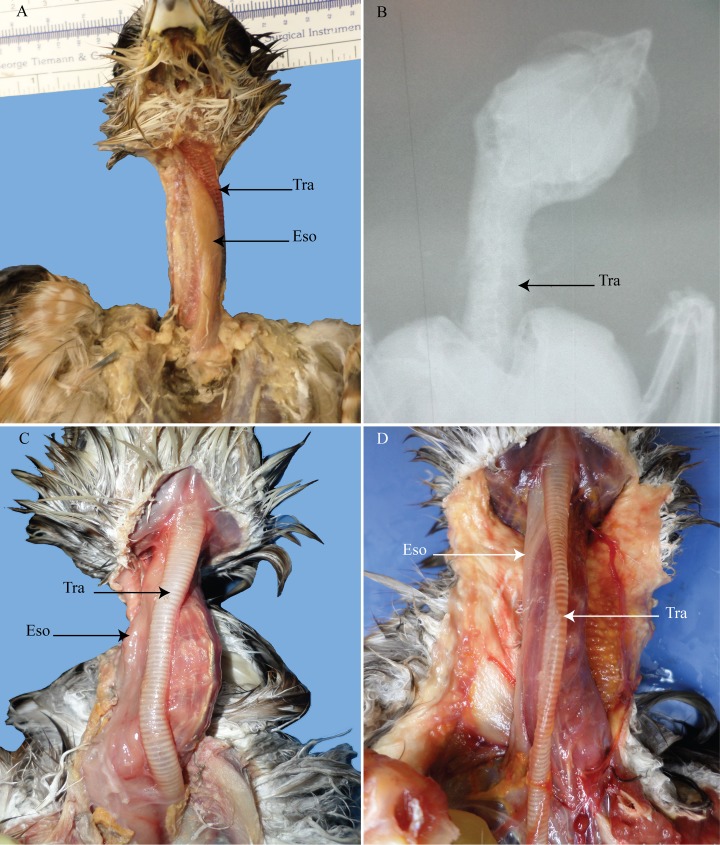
Accipitriformes. (A) *Accipiter cooperii* placement of the trachea and esophagus are on the left side of the neck. (B) radiograph of *A*. *cooperii* also showing the trachea on the left. (C) *Buteo jamaicensis* ventral view showing a more typical right lateralization. (D) *Buteo platypterus* ventral view displaying a midsagittal position of the trachea. The trachea is also twisted nearly 180°. Tra = trachea; Eso = esophagus.
